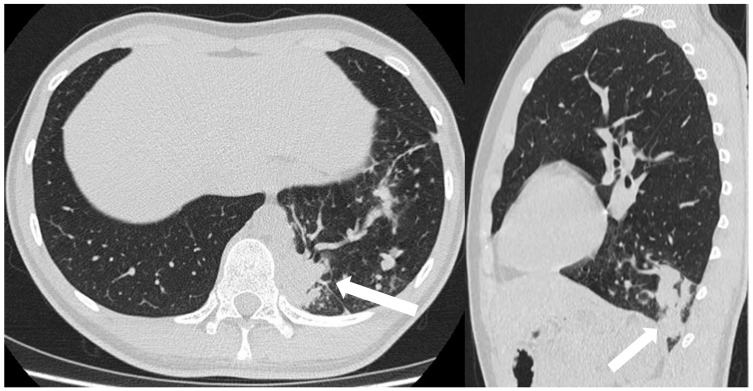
CT scans in axial and sagittal view of a 17 year old boy with suspected intra-lobar sequestration show a homogeneous soft tissue density mass within lung parenchyma (arrows).
